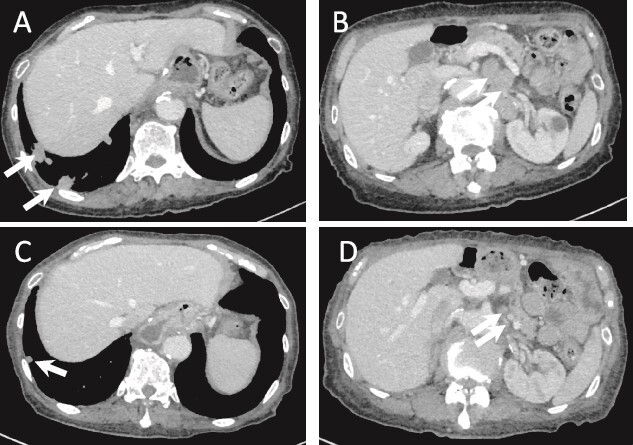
(a) CT image 5 months after surgery. Multiple lung metastases are seen (arrows). (B) Intra-abdominal lymph node metastasis is shown (arrows). (C, D) Lung and lymph node metastases after one cycle of chemotherapy show PR (arrows).
